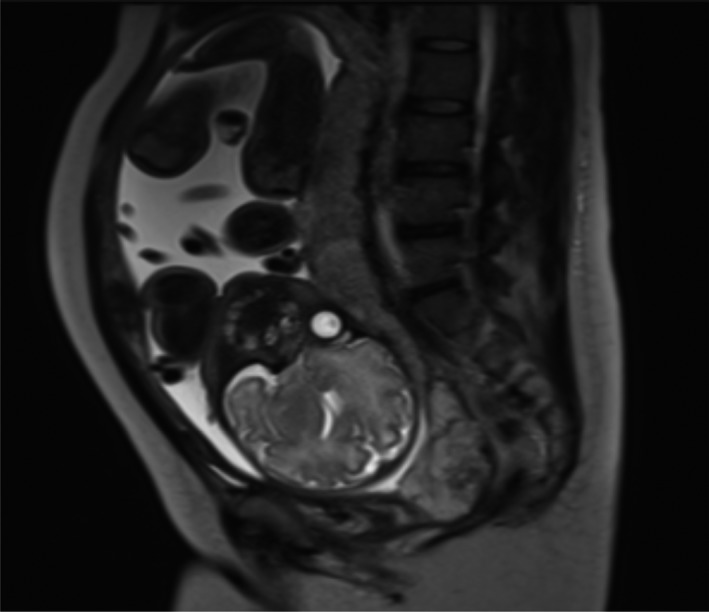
T2‐weighted sagittal MRI. Abnormality is demonstrated at the cervical area
